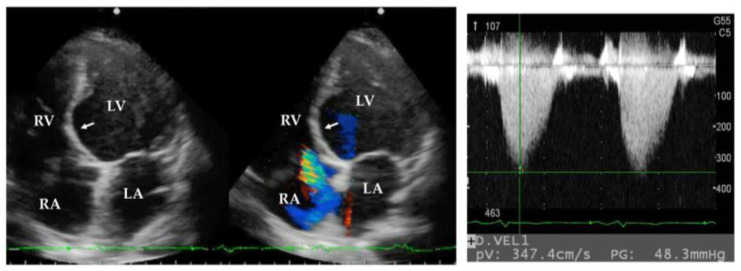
Transthoracic echocardiographic images of a patient with SAPH. Transthoracic echocardiography demonstrates a septal thinning (arrow) and regurgitant tricuspid jet, suggesting pulmonary hypertension in a patient with cardiac sarcoidosis. SAPH, sarcoidosis-associated pulmonary hypertension; RA, right atrium; RV, right ventricle; LA, left atrium; LV, left ventricle.
